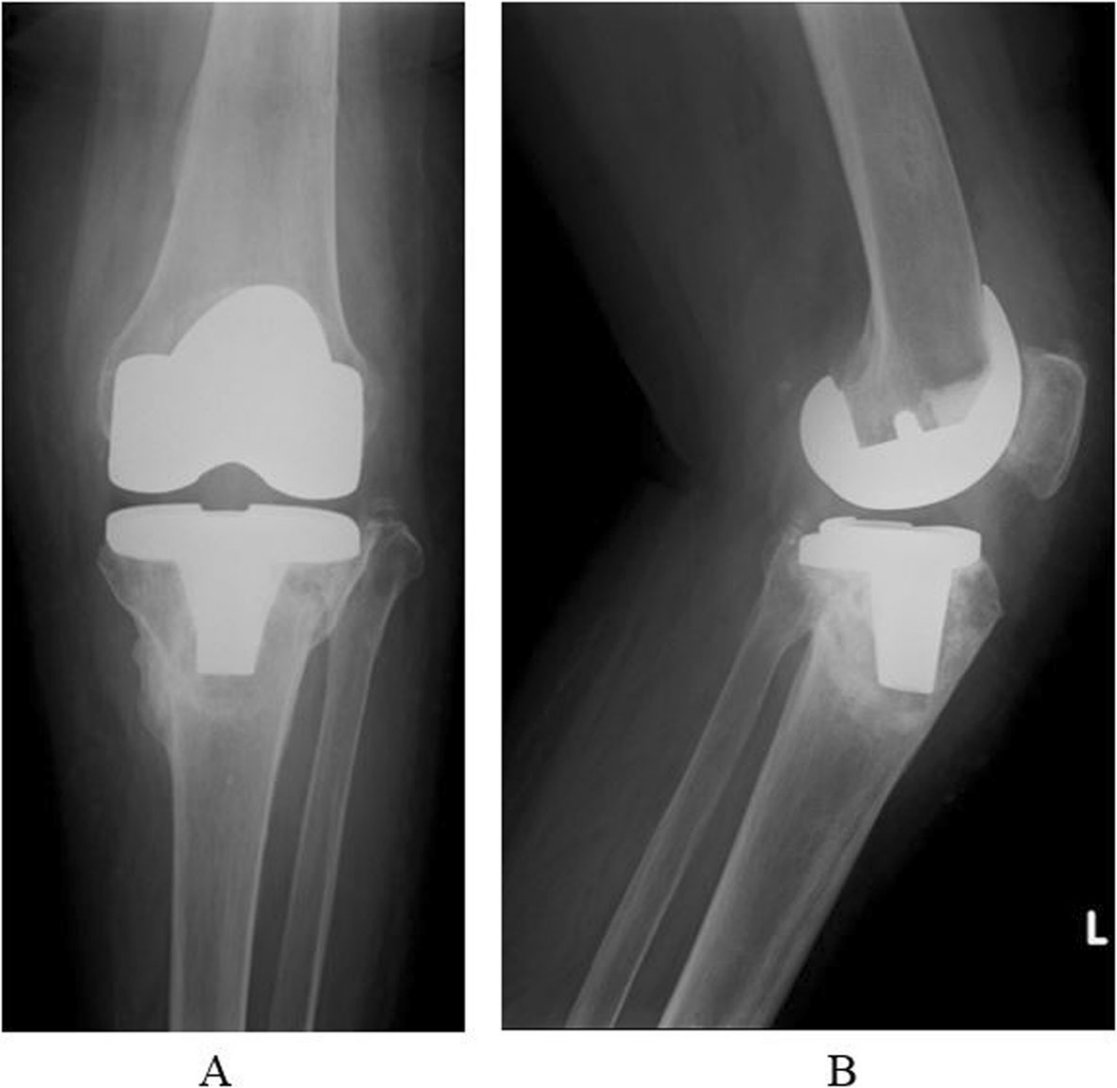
a After ring removal, antero-posterior X-ray. b After ring removal, lateral angle X-ray
